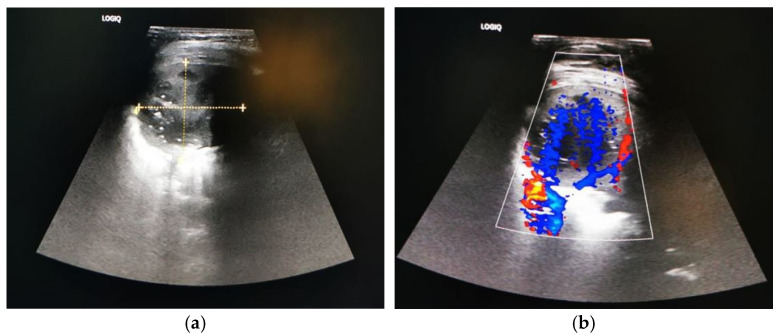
Two-year old male child with pulmonary abscess: thoracic ultrasonography showing (a) round–oval lesion with heterogeneous anechoic content, with no adjacent pleural effusion; (b) absent intrinsic vascular signal, but peripheral parietal vascular tract is present, suggestive of underlying congenital pulmonary airway malformation.
